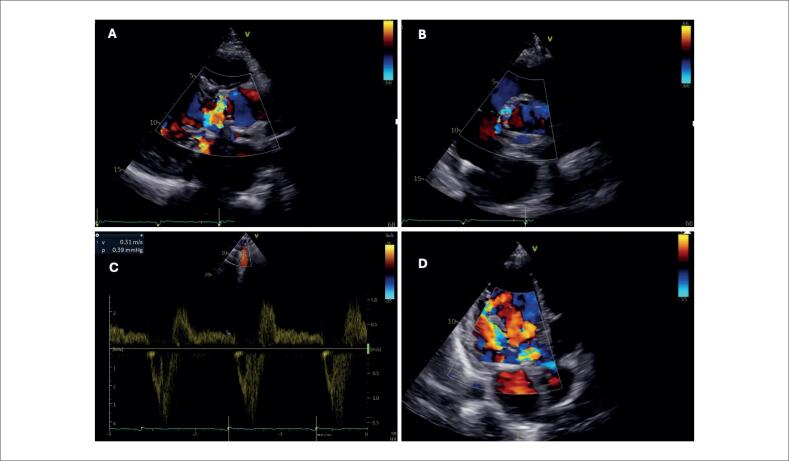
Ecocardiografia transtorácica demonstrando um jato regurgitante periprotético. A - Vista paraesternal de eixo longo. B - Vista de eixo curto. C - Doppler colorido mostrando inversão do fluxo holodiastólico no istmo aórtico, com velocidade terminal de 31 cm/s. D - Vista apical de três câmaras mostrando um jato regurgitante excêntrico que se estende por toda a cavidade ventricular esquerda.
